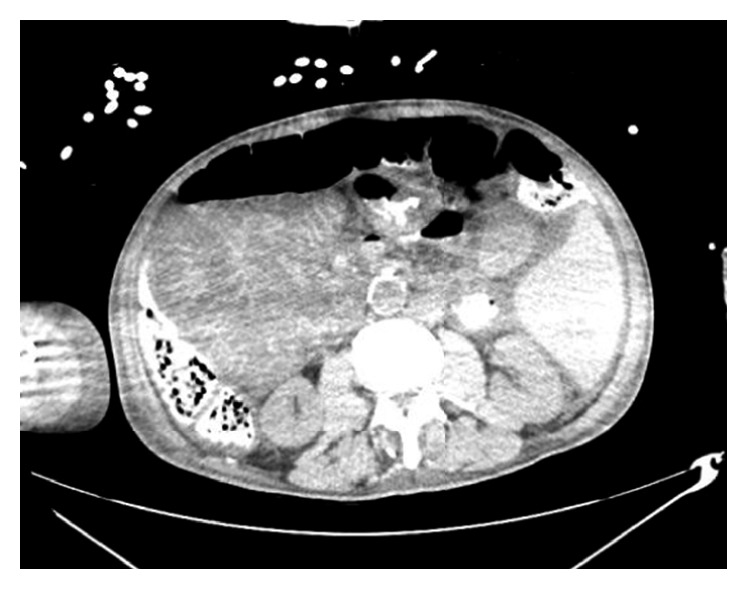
CT abdomen and pelvis showed prominent bowel loops with wall thickening, suggestive of bowel ischemia.
